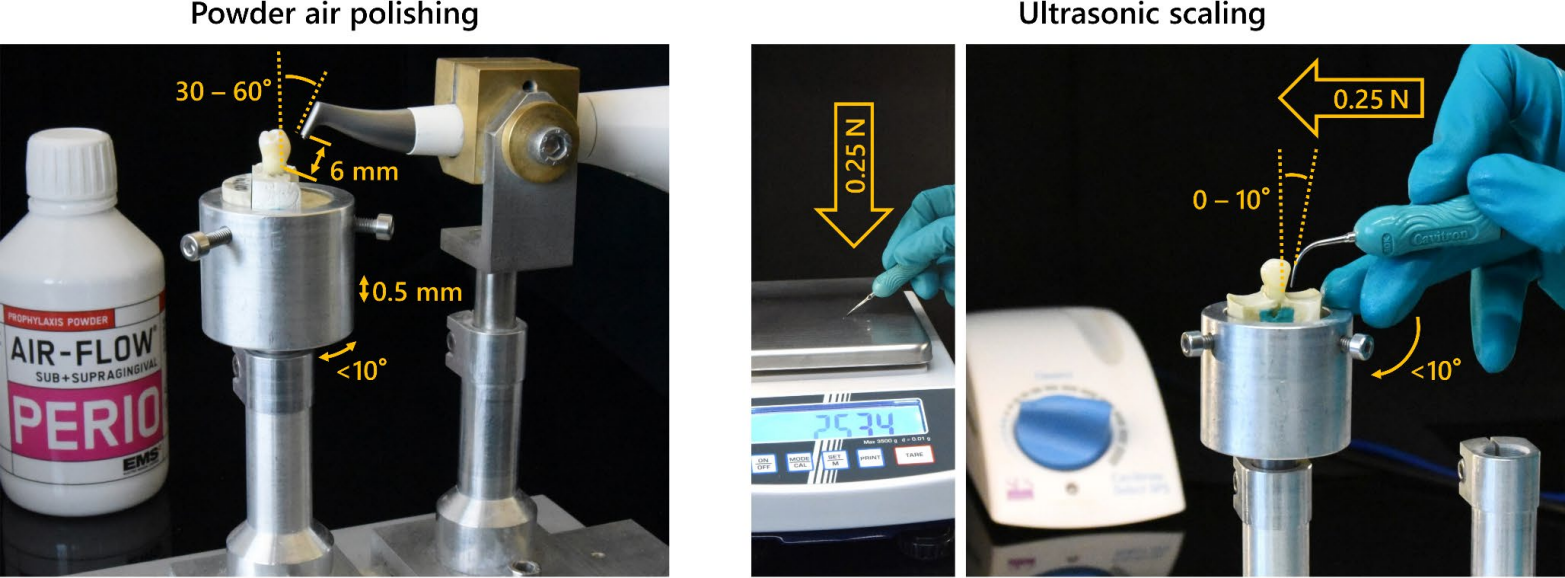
Visualization of the experimental setup for the prophylactic treatments with the corresponding distances, angles, rotational movements and manual calibration of the applied force, left: powder air polishing (Airflowhandy 3.0), right: ultrasonic scaling (Cavitron)
